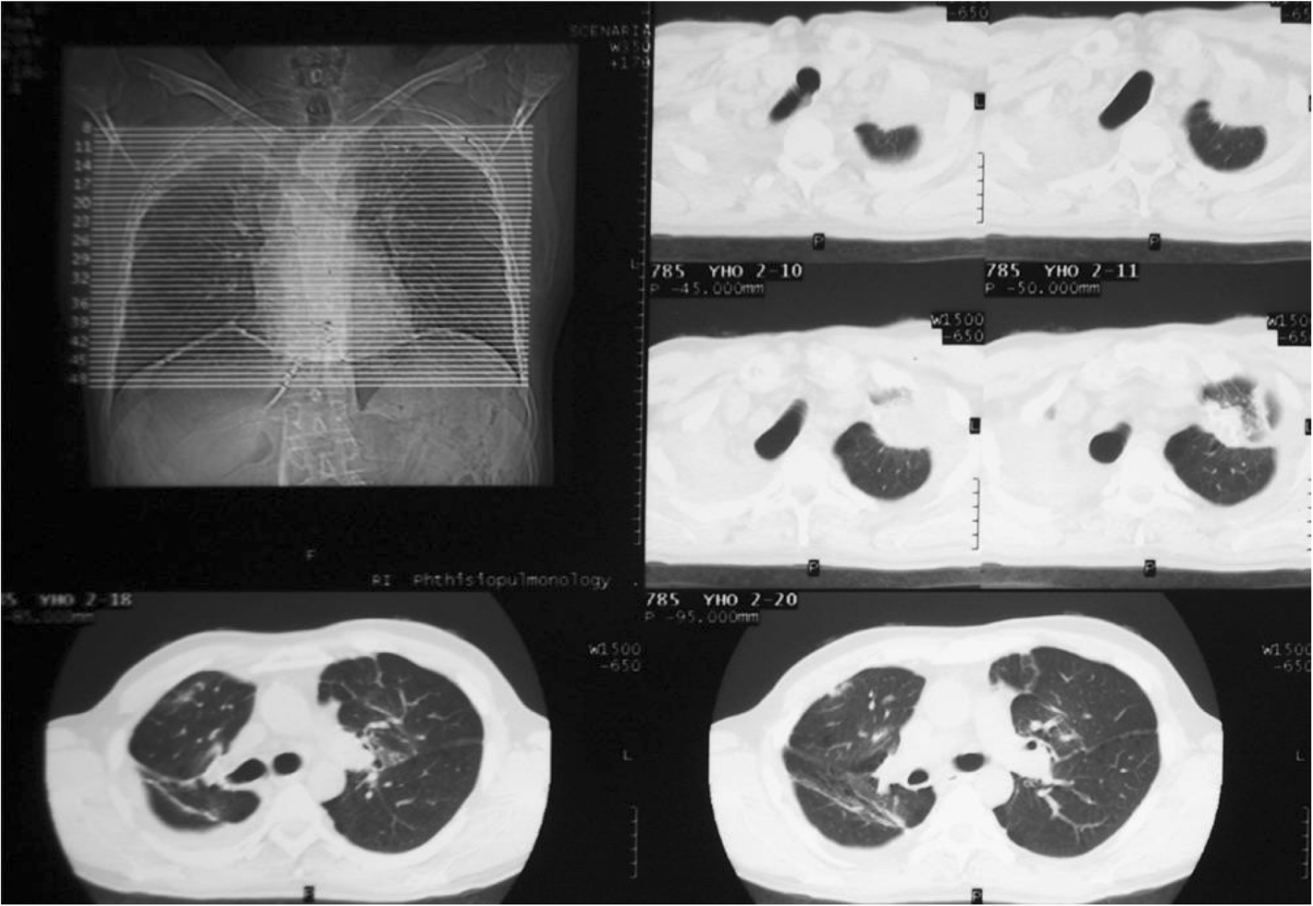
CT scan on discharge. Both operated lungs are extended
